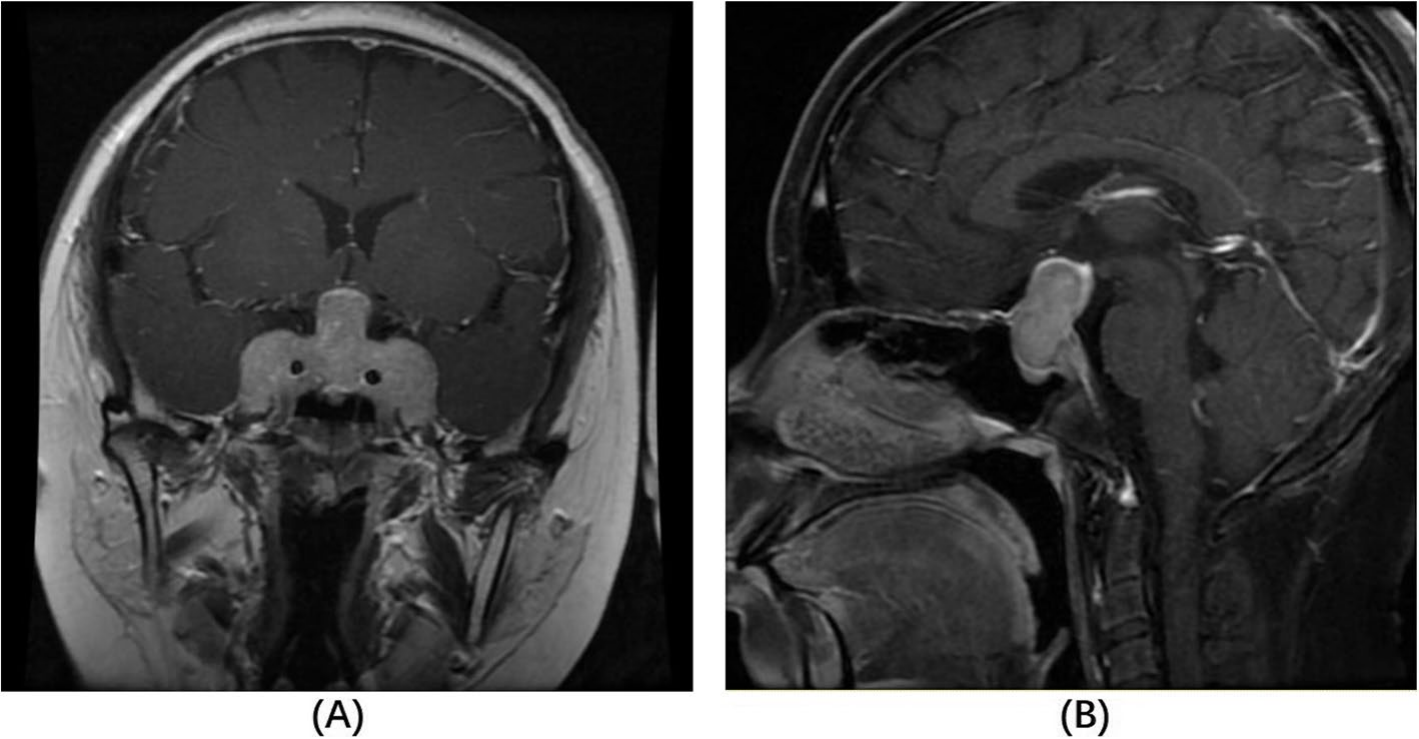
Contrast-enhanced coronal (A) and sagittal (B) T1-weighted MRI on admission. The sellar mass measured 51.8 × 29.3 × 14.0 cm (TD × VD × APD) with a heterogeneous density in the enhanced scan. The diaphragma sellea was dramatically elevated, with optic chiasm compressed. The sellar floor was sunken and bilateral cavernous sinus was surrounded (Knosp 4)
